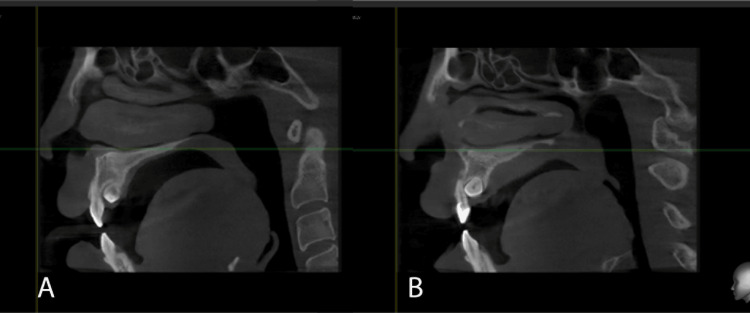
Pre-treatment CBCT CBCT: Cone-beam computed tomography A: Tooth 13; B: Tooth 23
